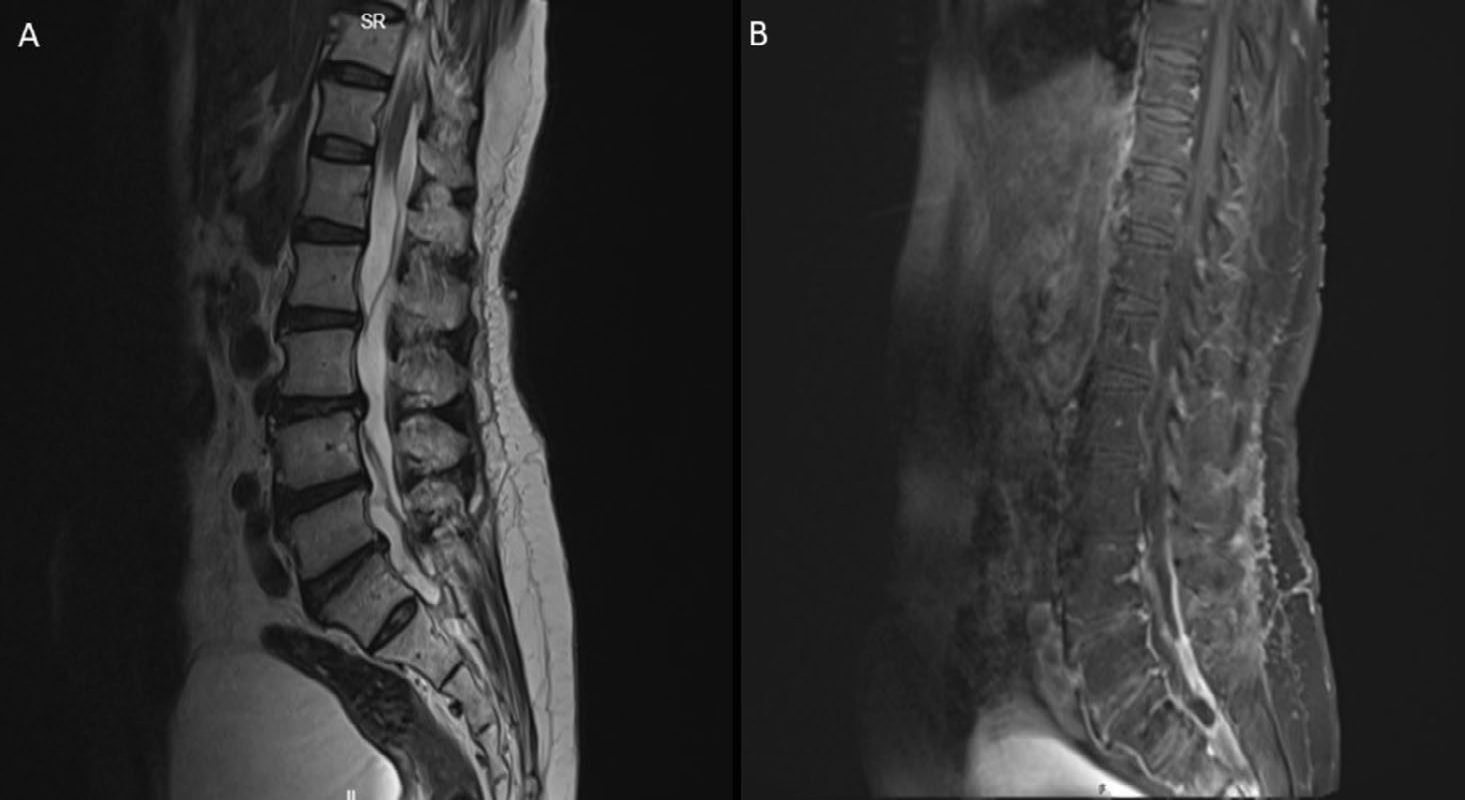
MRI of spine. T2 section showing a cyst at the cervico‐medullary junction.
